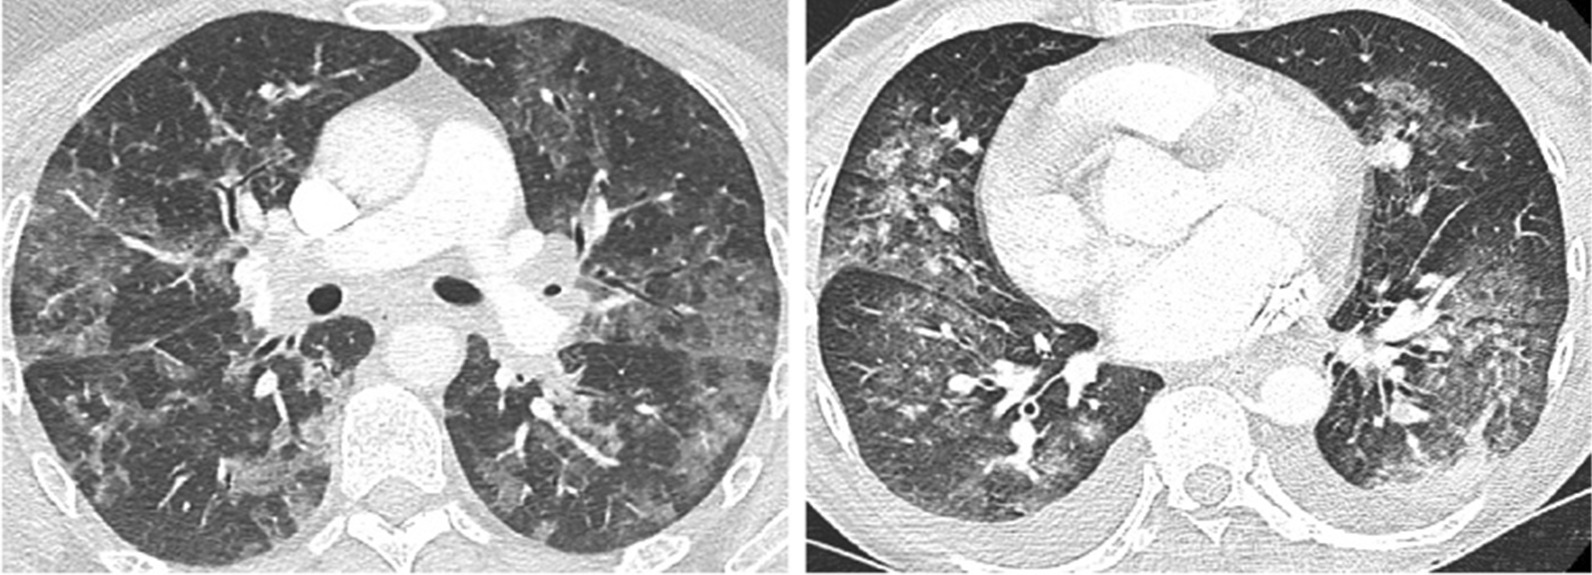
CT scan of two patients of 45-year and 48-years of age with influenza virus pneumonia and Goodpasture syndrome shows bilateral ground-glass opacities in contrast to COVD-19 patients (Hani et al. 2020)
